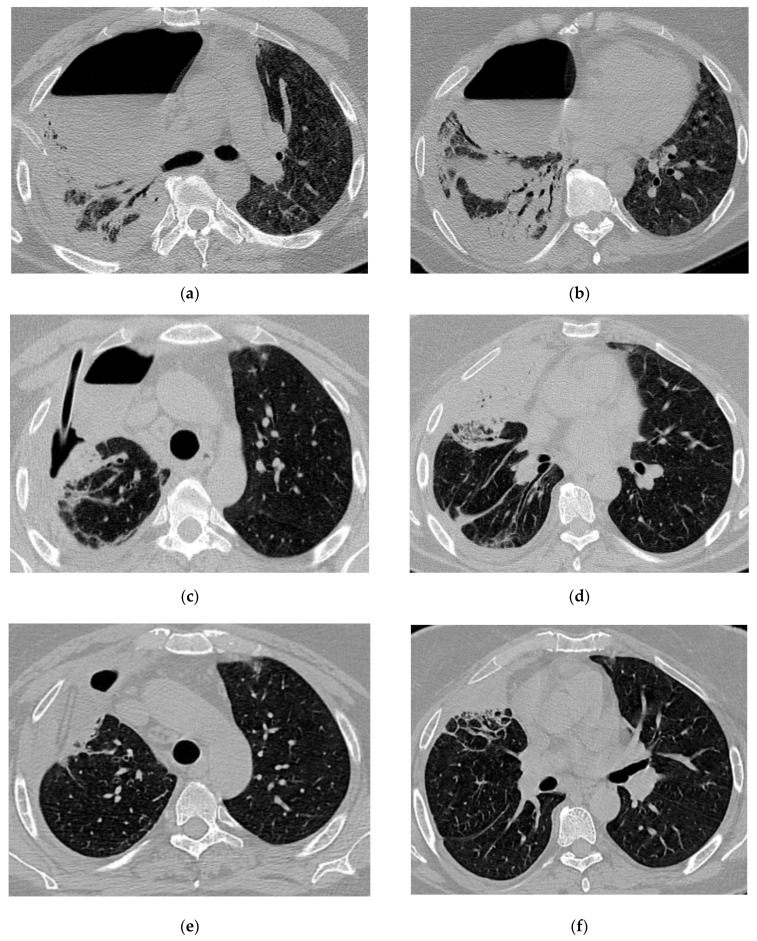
Radiologic evolution of case 3: Axial chest CT scans after first percutaneous ICD insertion revealing insufficient drainage of the purulent cavity; pleural effusion with few air inclusions; right ICD and diffuse interstitial infiltrates (a,b). In this case, multiple drainage and drain revisions were necessary to optimally control the purulent infection. Smaller right residual cavity with stable pleural effusion after ICD revision (c,d). Chest CT scans 1-month follow-up show the Foley catheter and decreasing size of the residual cavity (e). A follow-up CT scan after ICD removal showed small residual pleural and pulmonary fibrotic changes (f).
